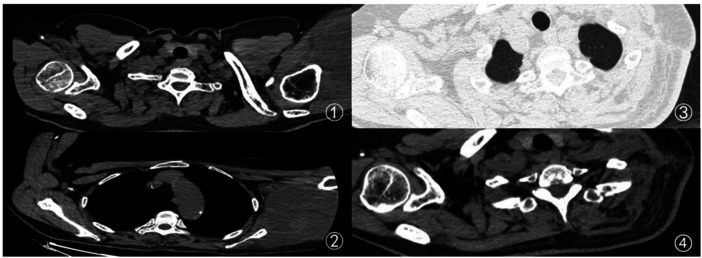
①: Preoperative 3-month CT scan of the patient, showing swelling of the left upper arm; ②: preoperative CT scan of the patient after completing 3 cycles of chemotherapy; ③: postoperative 6-month follow-up CT scan, with no tumor recurrence detected; ④: postoperative 1-year follow-up CT scan of the patient, with no tumor recurrence detected.
